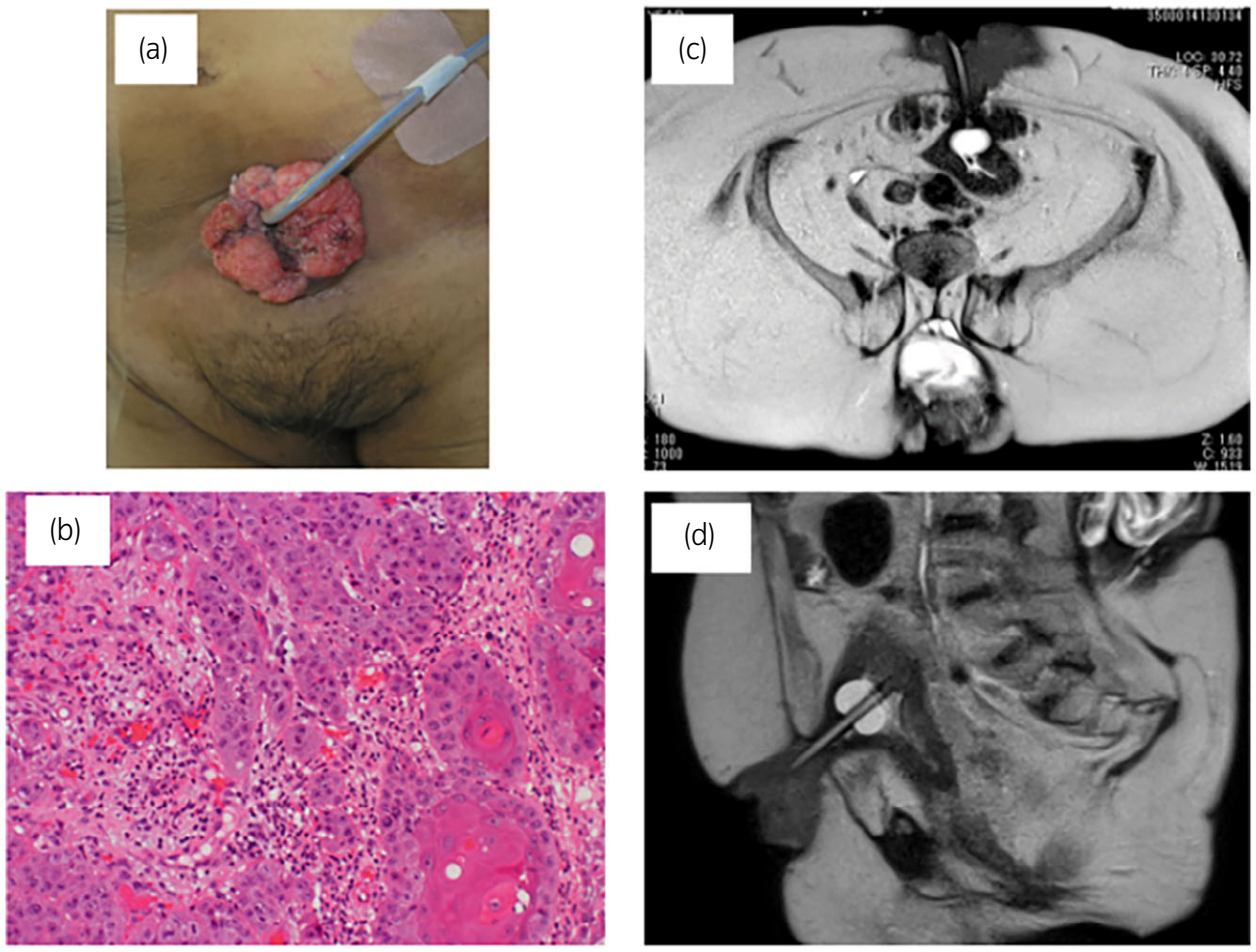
SCC arising from a SCT. (a) Clinical photograph showing an elevated lesion around the suprapubic cystostomy. (b) Tumor biopsy demonstrated a well‐differentiated SCC. (c, d). Magnetic resonance images showing that the tumor involved the bladder wall along the site of the catheter.
